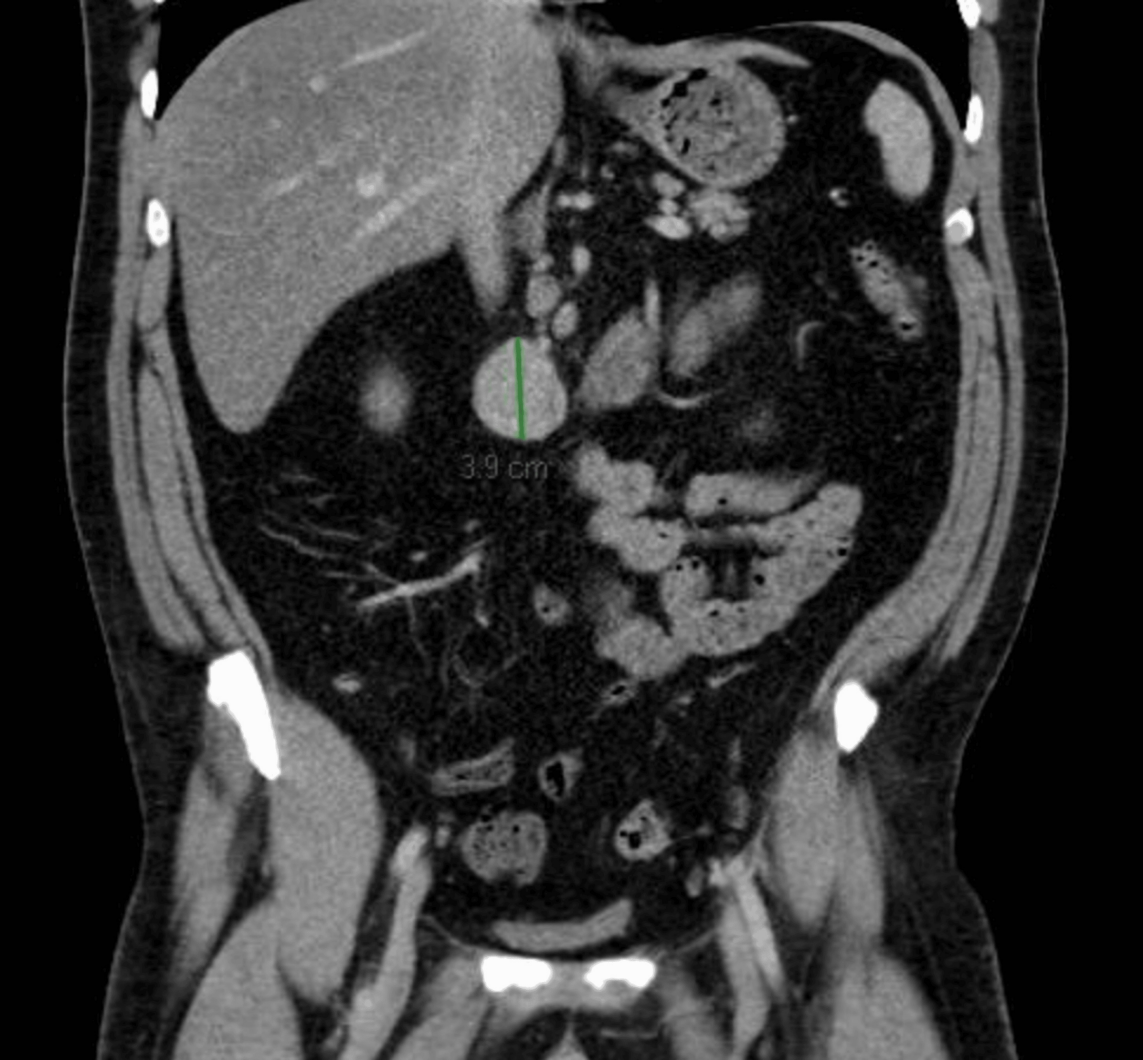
CT abdomen pelvis with IV contrast. Coronal view. The image shows a 3.9 cm craniocaudal measurement.
